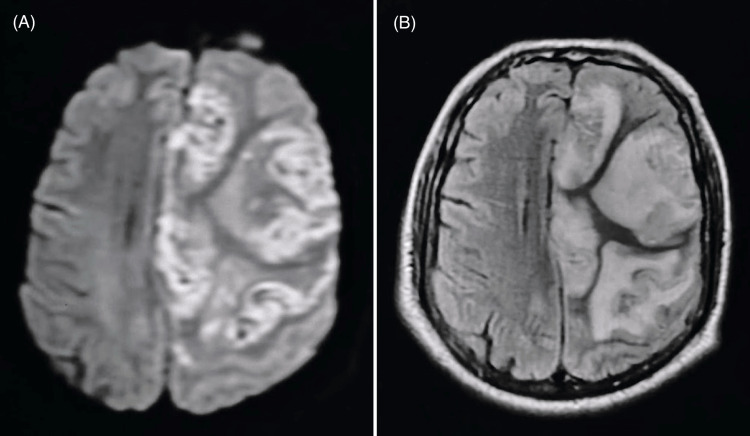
MRI of the brain showing hyperintense lesions in the left middle cerebral artery territory suggestive of acute infarcts. (A) Diffusion-weighted imaging (DWI) sequence. (B) Fluid-attenuated inversion recovery (FLAIR) sequence.
